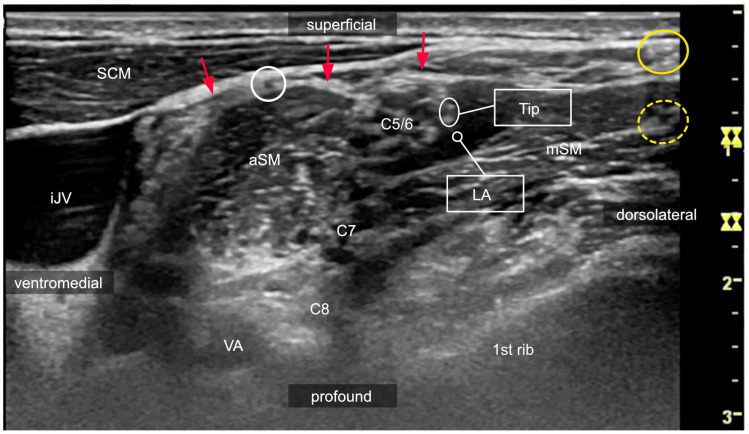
Ultrasound image captured at the level of the first rib, where spinal nerves C5 and C6 form the superior trunk, demonstrating the instant control of LA spread observation. The needle tip position can be controlled by the “double-dot sign”, which represents the orifice of the needle with the bevel up (white oval circle). In this patient, the out-of-plane approach was used to place a catheter in the direction of the plexus and sidestep the dorsal scapular nerve (yellow dotted circle), which could interfere with the in-plane approach from the posterior–lateral direction. LA injection can be stopped if one observes medial spreading toward the phrenic nerve (white circle). One branch of the supraclavicular nerve is marked by a yellow circle. If the supraclavicular nerves need to be blocked, the needle must be withdrawn and redirected because the prevertebral fascia (red arrows) represents a mechanical barrier for LA. aSM: anterior scalene muscle; mSM: middle scalene muscle; iJV: internal jugular vein; VA: vertebral artery; C7 and C8: ventral rami of the 7th and 8th spinal nerves, respectively. SCM: sternocleidomastoid muscle.
